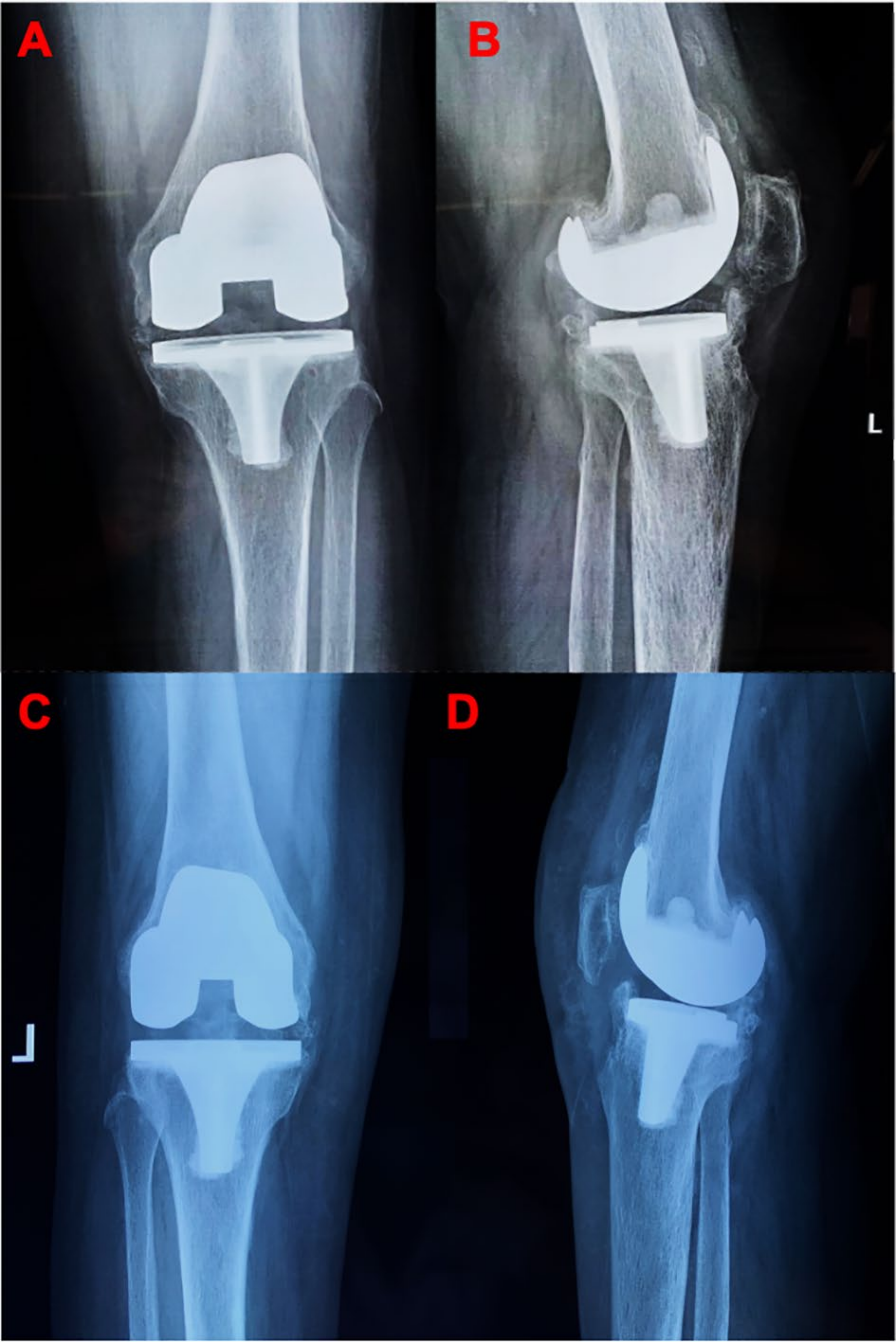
Postoperative TKR AP view (A) and lateral view (B) radiograph of the patient, showing type II HO (more than 2 cm of new bone formation). Postoperative TKR AP view (C) and lateral view (D) radiograph of patient accepted the arthroscopic debridement at the 3 months follow-up.
